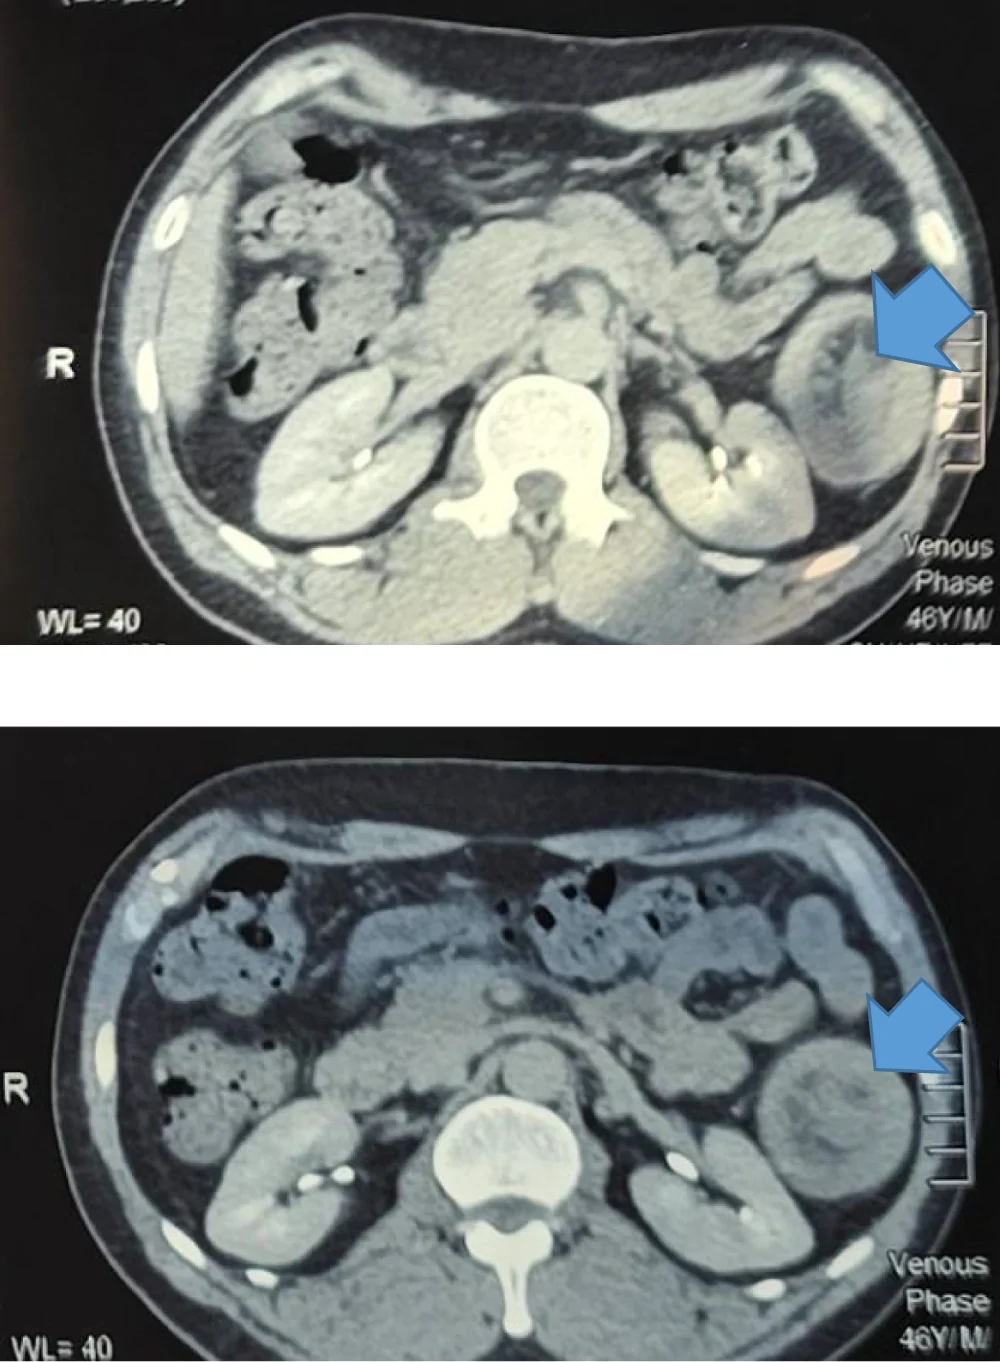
CT-scan: Transverse colon telescoping into splenic flexure and descending colon; fat-density mass measuring approximately 5 × 4 cm in descending colon; no bowel ischemia or perforation.
